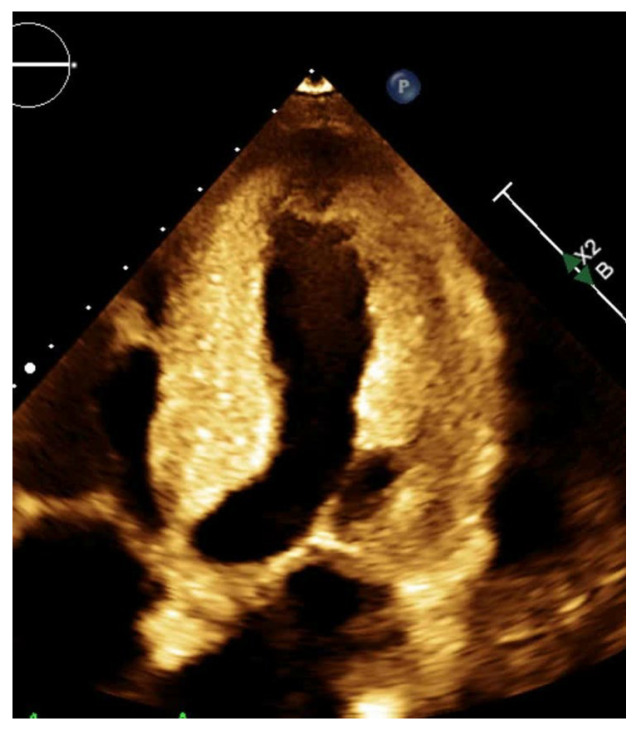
2D Echocardiography apical 4 chamber view depicting significant concentric LV hypertrophy with speckled or granular appearance in a patient with cardiac amyloidosis.
